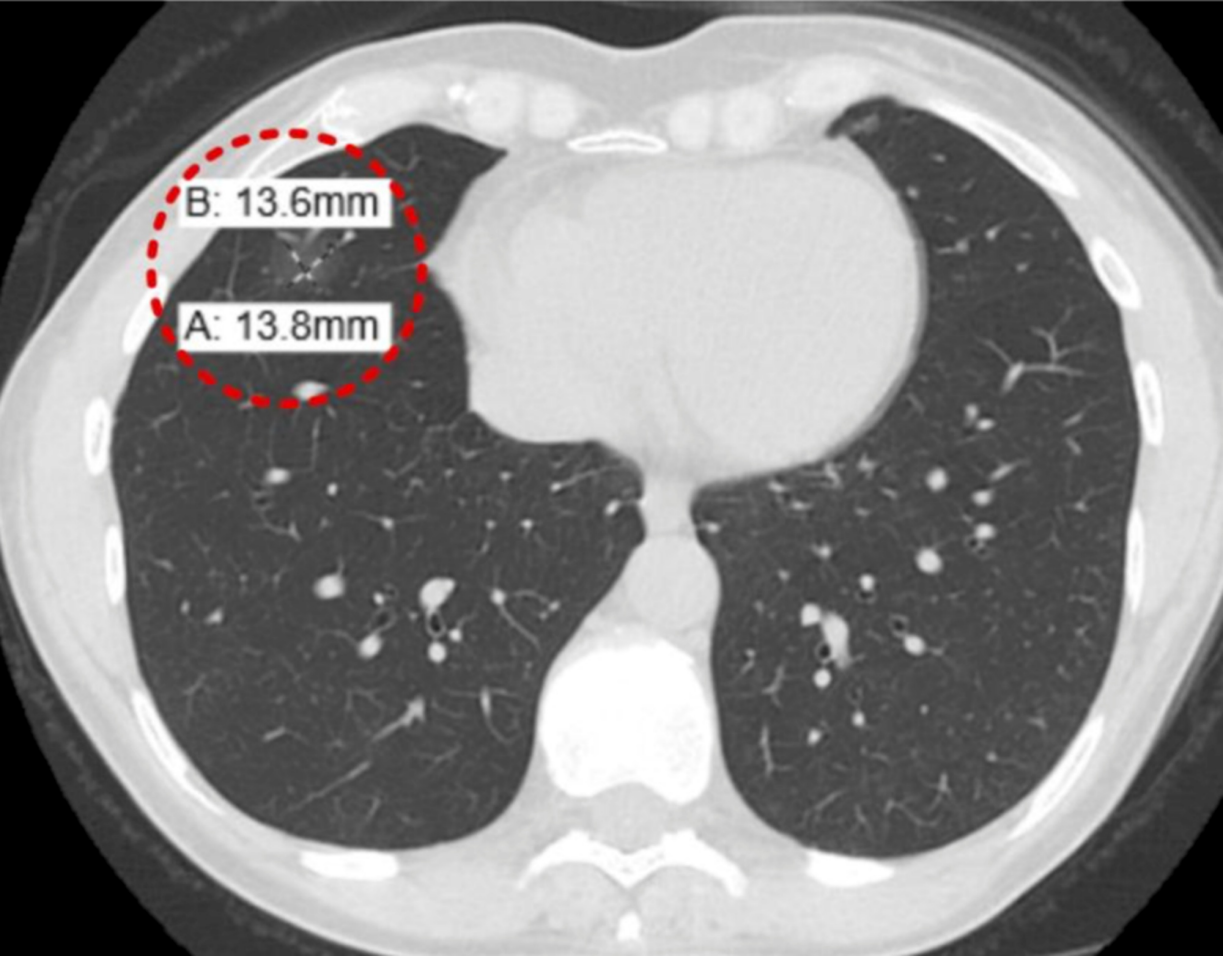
Non-contrast computerized tomography (NCCT) of the chest (10 months from previous CT) New 14 x 14 mm ground-glass opacity in the right lower lobe
